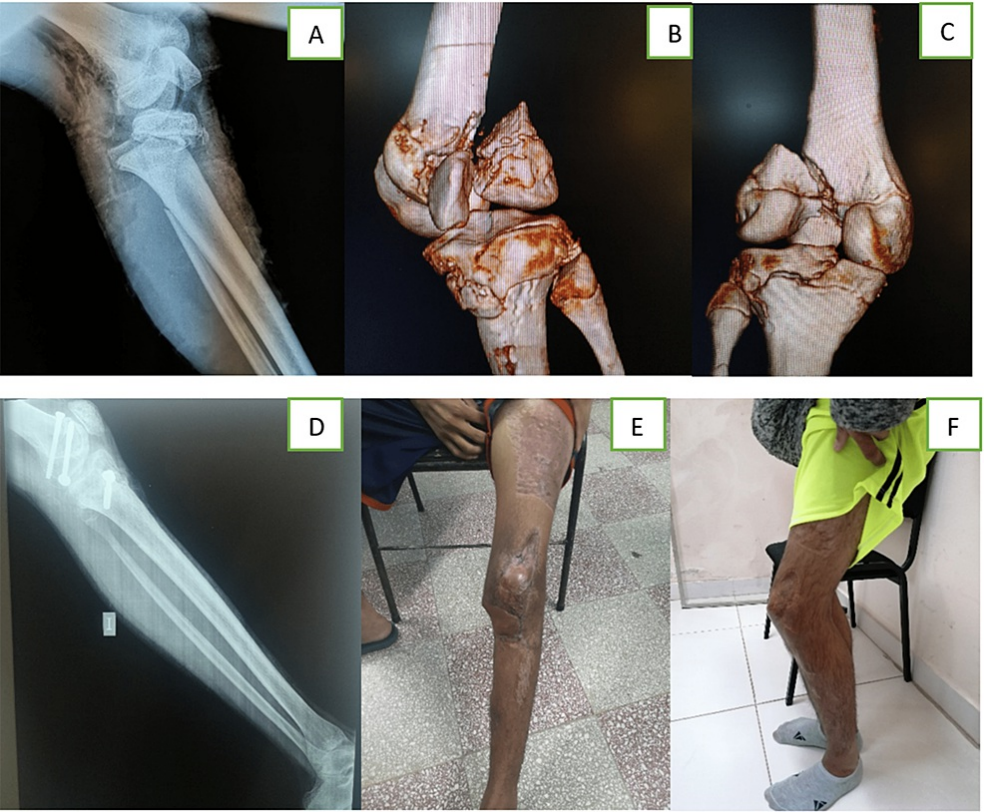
Clinical Case (Case 14) A, B, C: Salter-Harris Type IV femoral injury and Salter-Harris III tibial injury. D: immediate postoperative where both fractures managed by open reduction and internal fixation with lag screws. E and F: Three-month and 30-month follow-up showing satisfactory, but not perfect recovery. There is residual knee flexion. In the CT scans above, note the patella within the distal femur fracture.
